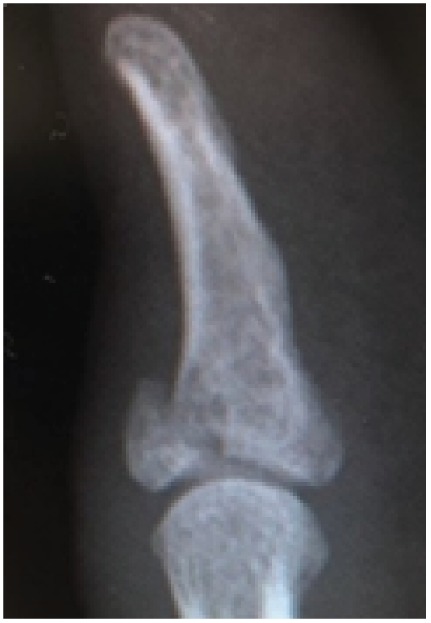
Lateral radiograph at the injury site.
